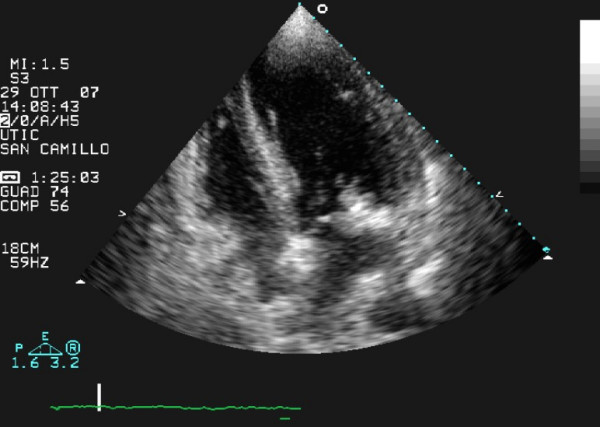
Two-dimensional echocardiogram, apical 4-chamber view: vegetation is seen attached to calcified mitral annulus.
